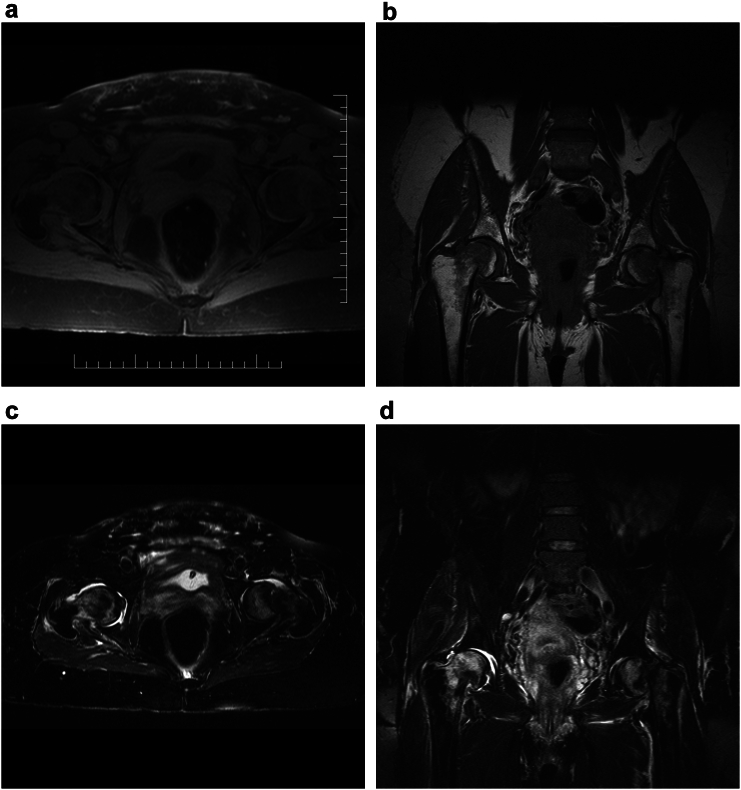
MRI of the affected hip joints in a 46-year-old woman performed 1 day postpartum. 2a, - Reduced signal intensity on T1-weighted transverse images. 2b, - Reduced signal intensity on T1-weighted coronal images. 2c, - Increased signal intensity on T2-weighted transverse images. 2d, - Increased signal intensity on T2-weighted coronal images.
